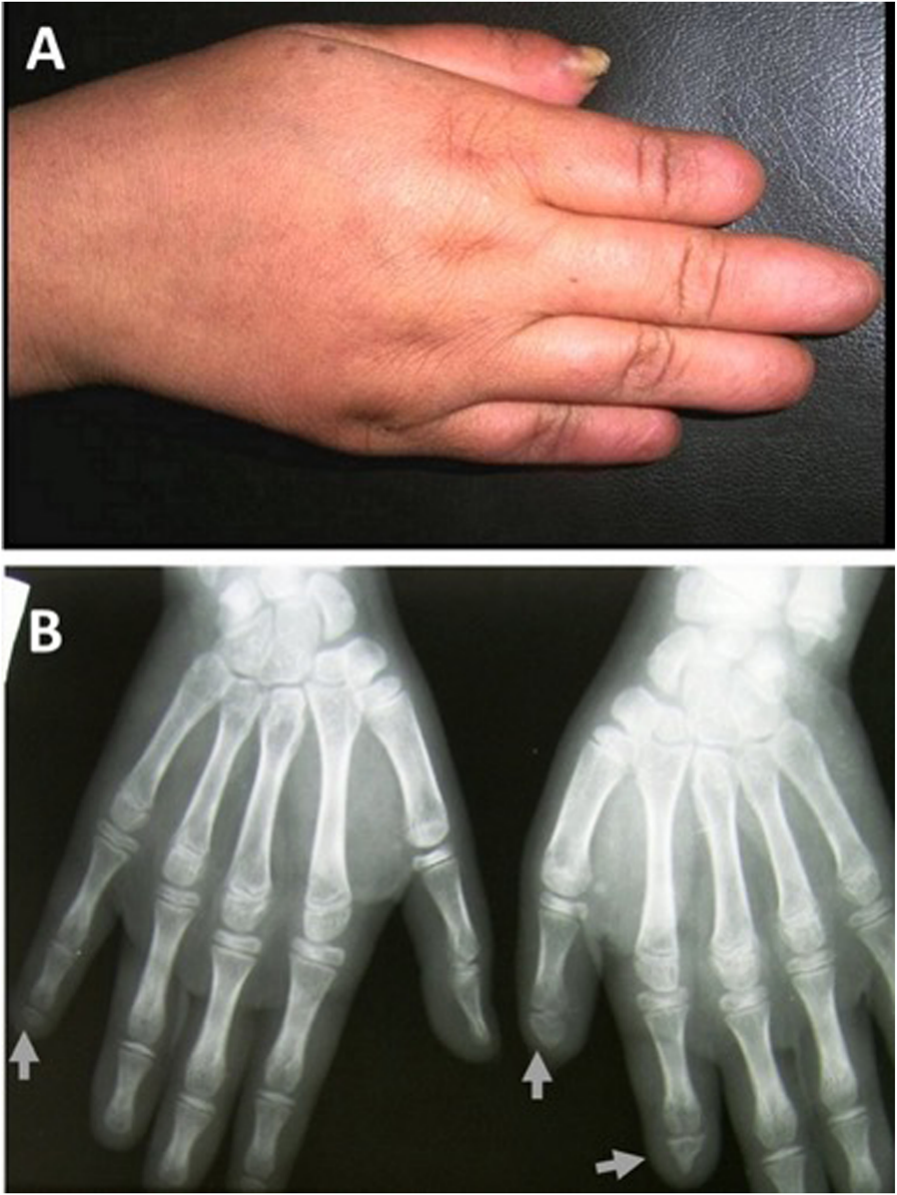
Shortening of terminal phalanges of fingers (a). Hand X-ray shows hypoplasia and/or complete absence of terminal phalanges (b) [2]
